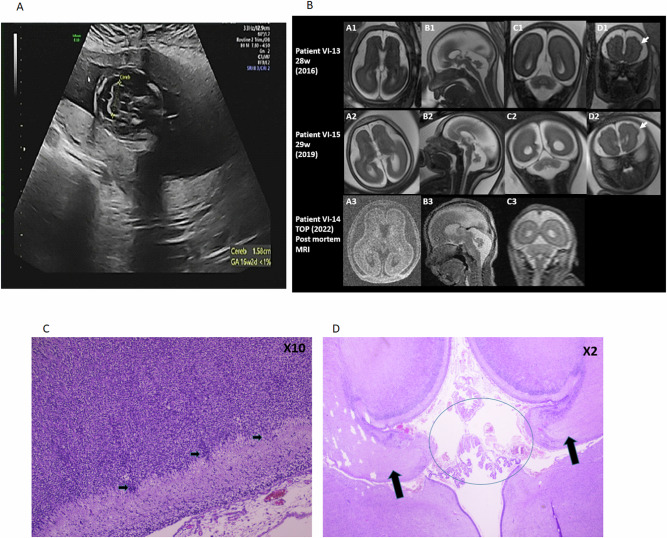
US, MRI, and pathological analysis of affected fetuses. A Ultrasound of brain at 19 weeks of VI13 demonstrating hypoplastic cerebellum (measurement between two calipers +) and enlarged cisterna magna. B MRI images of the three fetuses studied (VI13, VI14, VI15): axial T2 (A1, A2) and axial T1 (A3)—flat open sylvian fissure with small frontal lobes. Sagittal T2 (B1–B3)—dysmorphic corpus callosum (short and thick). Abnormal brain stem with flat pons. Abnormal small vermis, open fastigium, no primary fissure. Coronal T2—small TCD (C1–C3), abnormal gyral pattern (arrow) (D1, D2). C Affected TAF8 deficiency, fetal brain, showing leptomeningeal glioneuronal heterotopia (arrows), magnification—×10. D Demonstrating partial agenesis of the CC (circle) and intact parts of the CC (arrows), magnification—×2.
